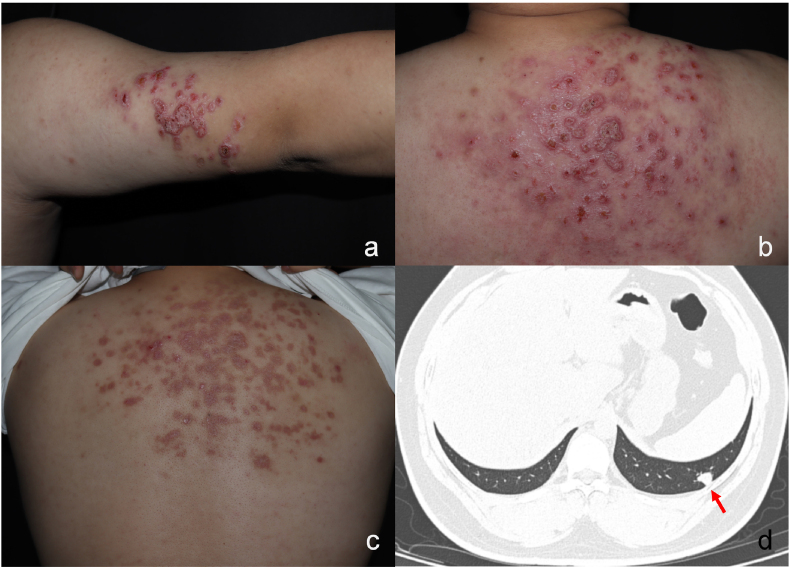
Clinical and imaging presentations of this patient a, b: multiple erythematous plaques with signs of erosion, scabbing, and exudation were observed c: Skin lesions after 6 months of voriconazole treatment, showing improvements; d: Solitary pulmonary nodule found by chest CT with no obvious pulmonary symptoms at presentation.
